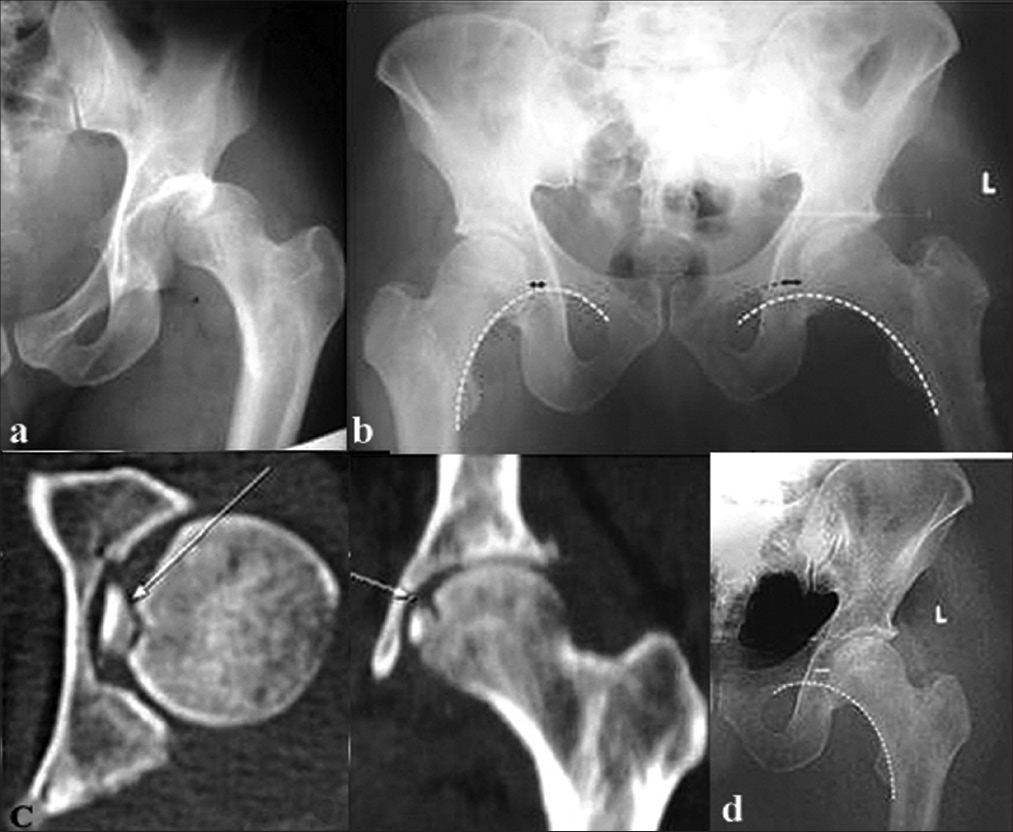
X-ray of left hip joint (an anteroposterior view) in a 53-year-old male showing (a) Posterior dislocation of hip joint. (b) Postreduction X-ray shows noncongruent reduction: a broken Shenton’s line and increase in medial joint space. (c) CT scan showed a noncongruent reduction due to the presence of a large osteochondral fragment from the femoral head. (d) The fragment was excised and the joint reduced, leading to congruent reduction
